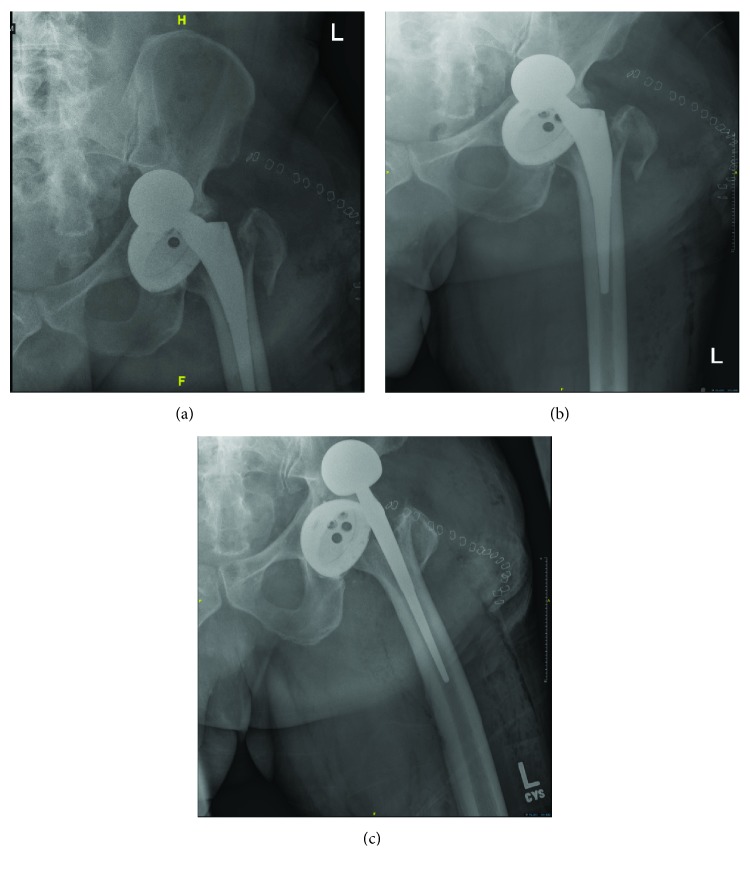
AP and lateral X-ray of the left hip 1 postoperative day after insertion of implants demonstrating anterior dislocation of left femoral head prosthesis and fracture of the greater trochanter.
